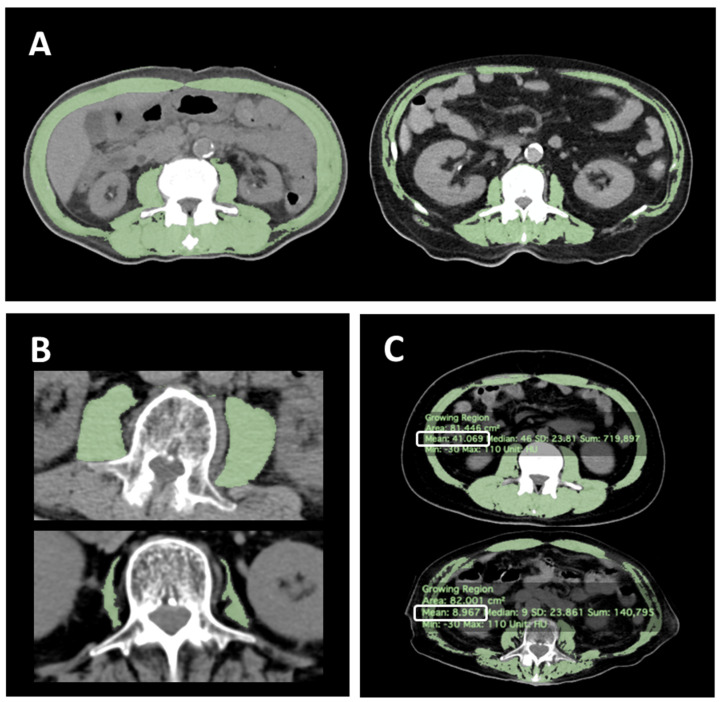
Representative CT images at the level of the third lumbar vertebra. The CT images of patients with (A) (left) high and (right) low L3-SMI and (B) (above) high and (below) low L3-PMI. The areas in green represent the region of skeletal muscle automatically annotated by OsiriX DICOM viewer (version 12.0.3; Pixmeo SARL, Switzerland). (C) The images of the skeletal muscles with (above) high and (below) low values of L3-MRA. The numbers in white squares denote the L3-MRA values that present the average density values (HU) of the skeletal muscle mass at the L3 level. CT, computed tomography; L3-SMI, skeletal muscle mass index at the level of the third lumbar vertebra; L3-PMI, psoas muscle mass index at the level of the third lumbar vertebra; HU, Hounsfield Units; and L3-MRA, muscle radiation attenuation at the level of the third lumbar vertebra.
